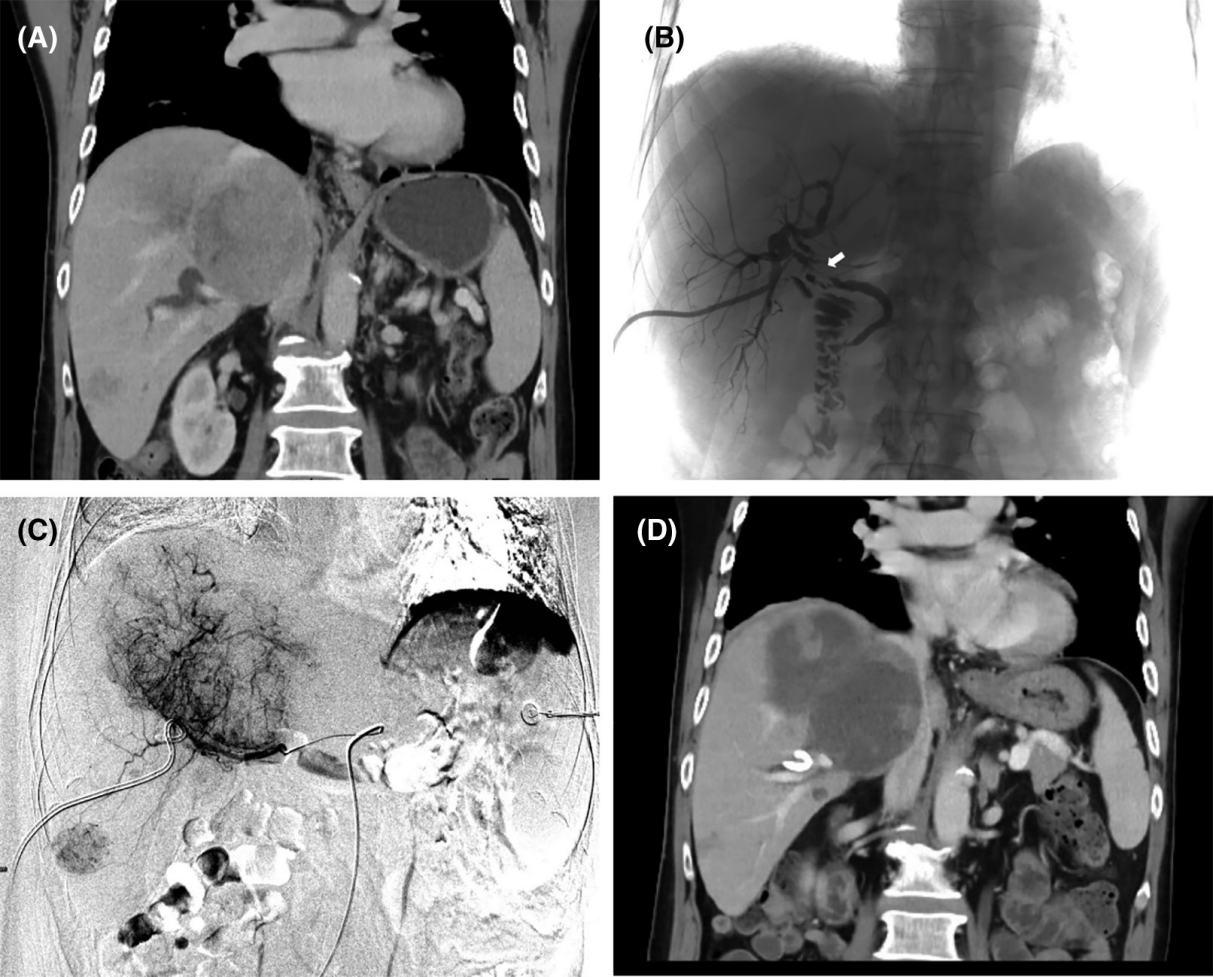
A 67‐year‐old man with B1‐BDI unresectable hepatocellular carcinoma: (A) Enhanced CT at diagnosis shows multiple nodular lesions and bile duct dilation in liver; (B) Percutaneous transhepatic cholangial drainage (PTCD) was performed with one external drainage tubes. Under digital subtraction angiography monitoring, imaging showed a filling defect inside the bile duct (white arrow); (C) The diagnostic arteriography revealed hypervascular lesions during the first DEB‐TACE procedure; (D) Enhanced CT at 4 weeks after successful drainage by PTCD and sequential DEB‐TACE shows obvious necrosis in the intrahepatic tumors. cTACE, conventional transarterial chemoembolization; DEB‐TACE, drug‐eluting beads transarterial chemoembolization.
